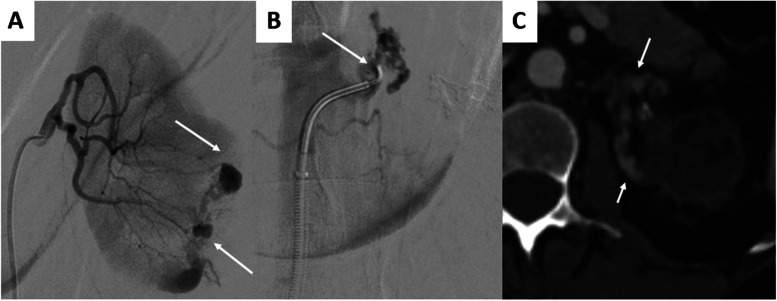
A 76-year-old patient presented with left lumbar pain and was found to have a large angiomyolipoma with active bleeding. A Angiography revealed two distinct bleeding sites from two different branches of the left renal artery (arrows). B After embolization of the lower branch with NBCA/Lipiodol (ratio 1/1), an attempt to catheterize the upper branch was performed, but an arterial dissection of renal arterial appeared (arrow). C The CT scan in axial section at arterial phase performed two years later shows atrophy of the upper pole of the left kidney (arrows)
